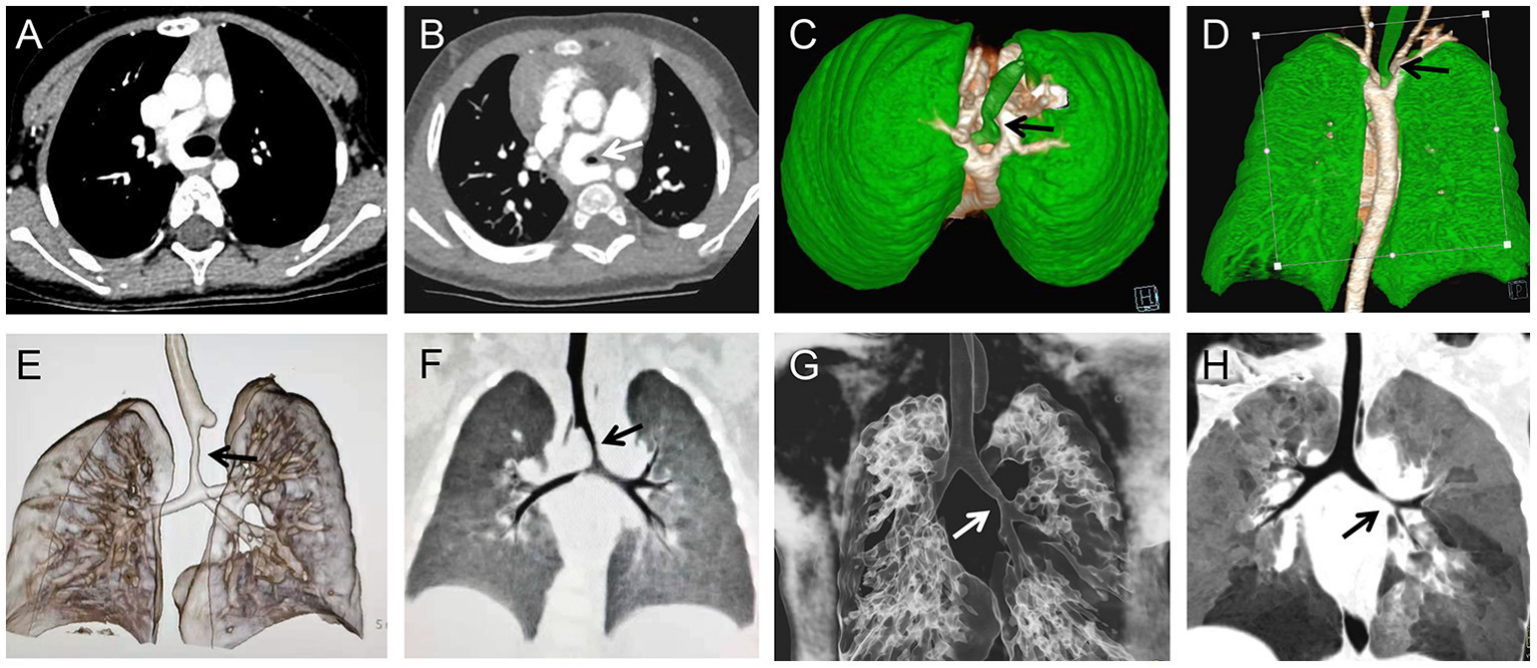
Pulmonary CT and three-dimensional reconstruction of the airway, preoperatively showing the airway stenosis (arrows) was caused by pulmonary artery sling (A,B), double aortic arch (C,D), and a representative image of the stenosis (arrows) located in the trachea (E,F) and bronchus (G,H).
